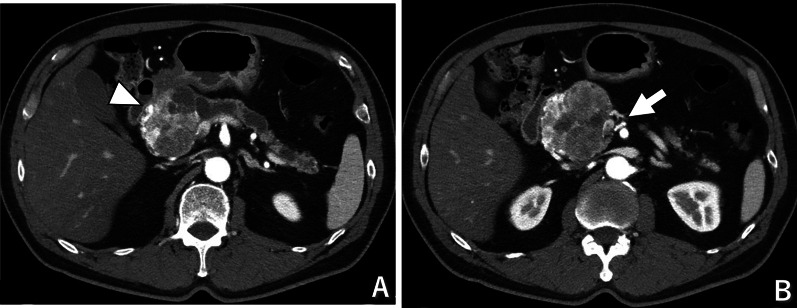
Enhanced computed tomography findings. A An 8-cm tumor was located at the head of the pancreas, composed of numerous tiny cysts with abundant vascularization. The main pancreatic duct was obstructed by the tumor, and the distal pancreatic duct was dilated. The arrowhead shows the gastroduodenal artery, a feeding artery of the tumor. B The dorsal pancreatic artery, branching from the superior mesenteric artery, was another feeder of the tumor (arrow)
